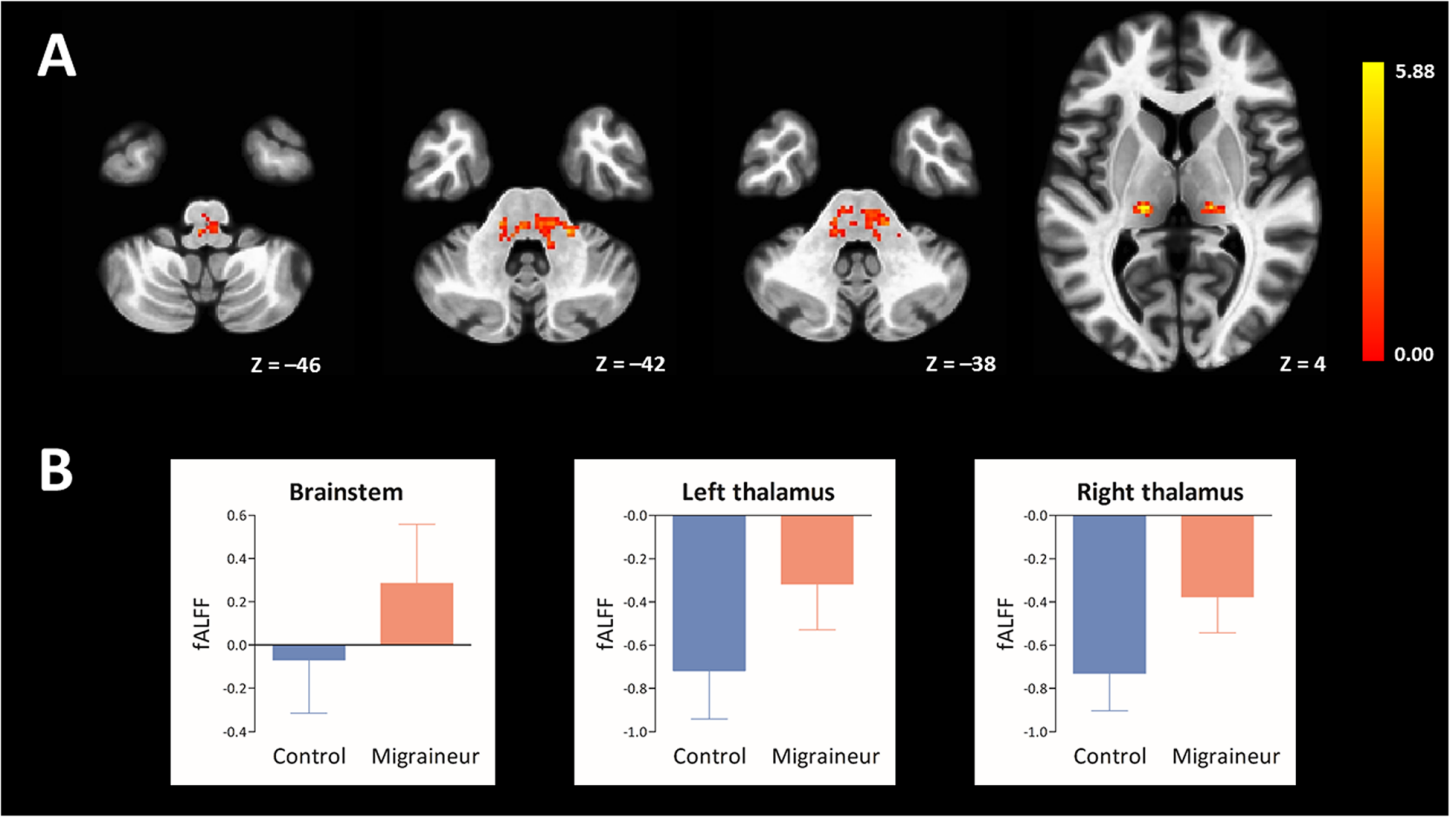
Brain regions of significant fALFF increases in migraine patients without aura compared to matched controls are rendered on the axial template images of the standard MNI brain (A). The left side of each MR image is the left side of the brain. The color bar indicates *t*-statistics. Mean (bars) and standard deviation (whiskers) for the fALFF of the brainstem and bilateral thalamic clusters in 31 controls and 44 migraine patients (two-sample *t*-test, all *p* < 0.001) (B)
